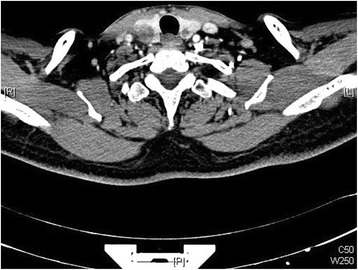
Thyroid metastases of case 1. Coronal computed tomography scan revealed bilateral solid nodules in the thyroid gland
